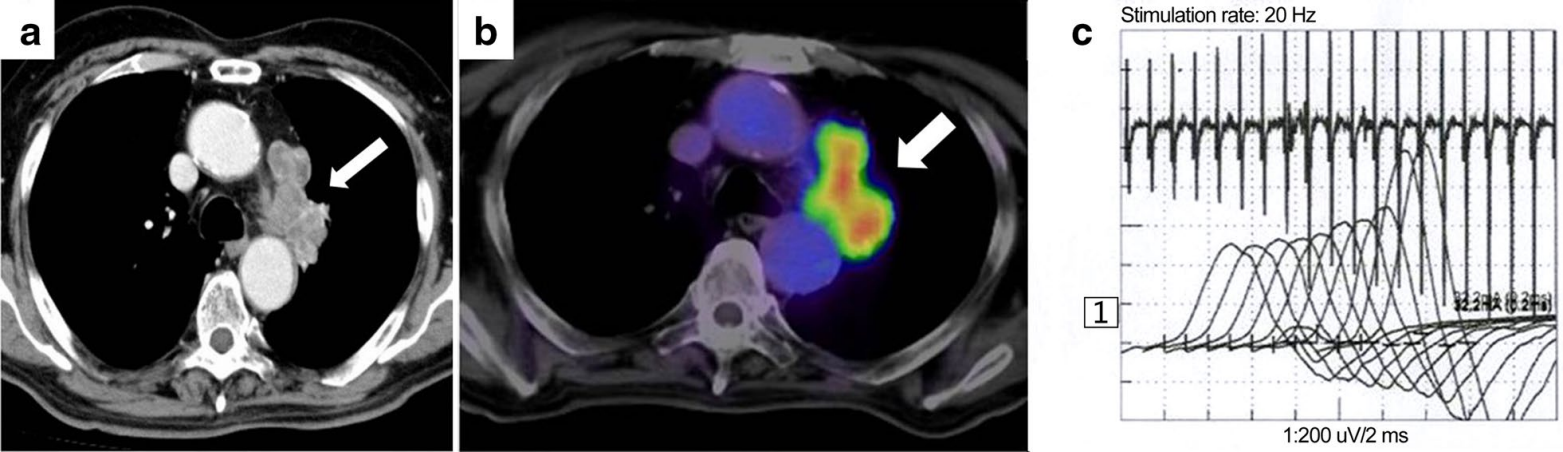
Radiological findings and electromyography in the SCLC patient with LEMS at the age of 85 years. a Chest contrast CT revealed mediastinal and pulmonary hilar lymphadenopathy (*arrow*) on March 10, 2015. B, 2-deoxy-2-[18F]fluoro-d-glucose (FDG) positron emission tomography (PET)-CT showed FDG accumulation in the mediastinal and pulmonary hilar lymph nodes (*arrow*) on March 24, 2015. c Electromyography following stimulation at 20 Hz showed waxing on April 9, 2015
